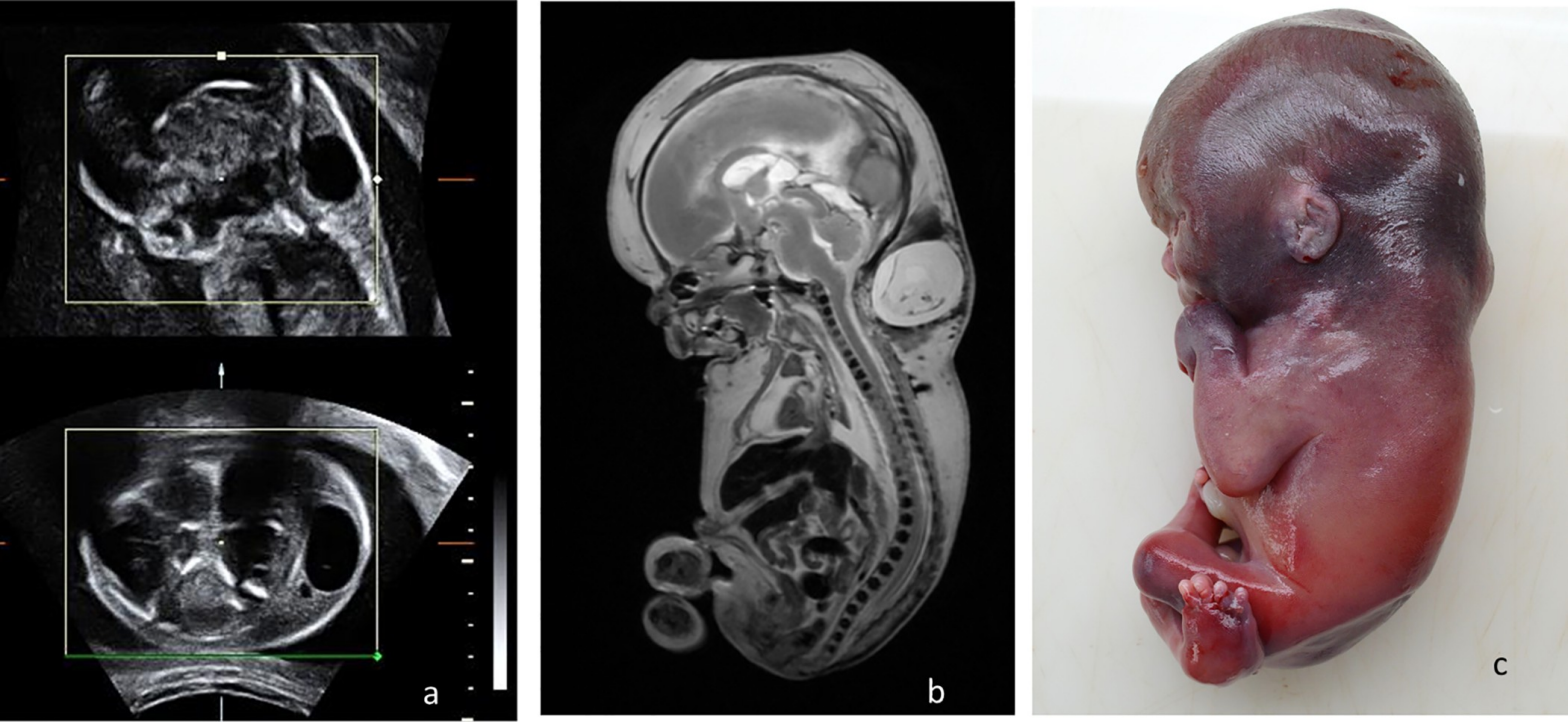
23 weeks of gestation plurimalformed fetus diagnosed prenatally with 18 trisomy. a) prenatal ultrasonography; b) pm-MRI at 3 T, T2 WI sagittal section, demonstrating hydrops fetalis, hygroma, partial corpus callosum agenesis and limbs malformations; c) macroscopic examination of the fetus.
